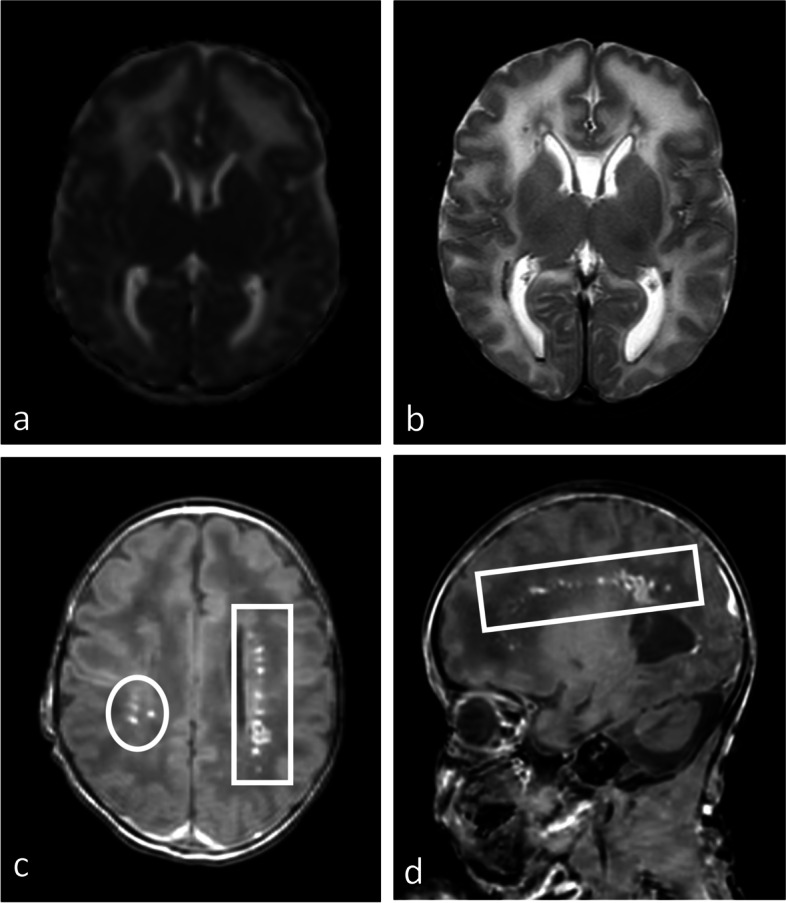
Postnatal MRI of the brain at day 19. a Apparent diffusion coefficient (ADC) map in the axial plane. b T2-weighted axial image. c T1-weighted axial plane. d T1-weighted sagittal plane. a, b Diffuse white matter signal alterations on T2 weighted images with increased ADC values on diffusion weighted imaging. c, d Extensive bilateral periventricular calcifications in the axial and sagittal plane (circle and square)
